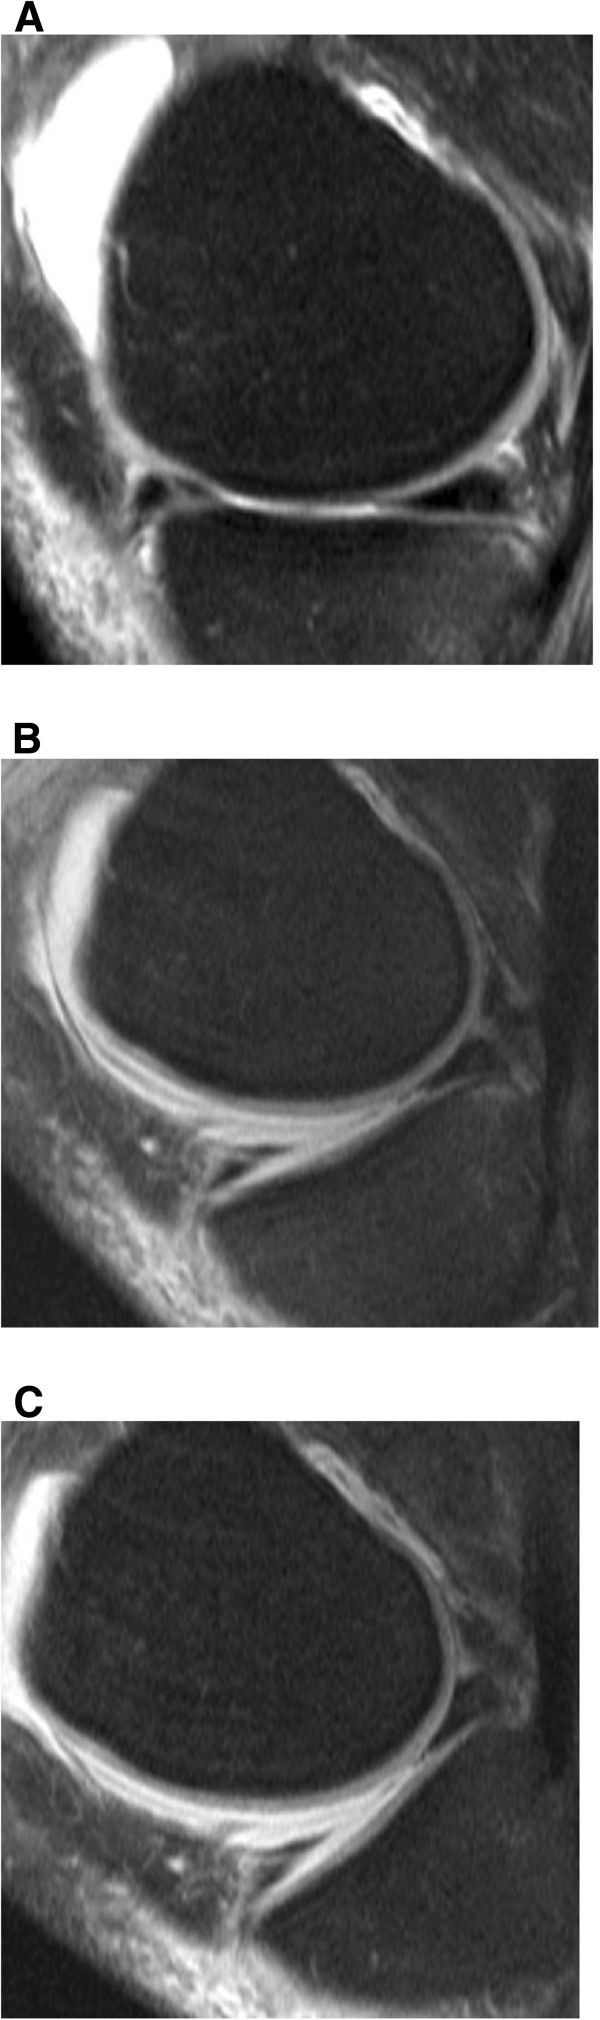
Oblique vertical tear of the anterior horn of the medial meniscus. T2-weighted TSE FS sagittal image (2900/90) obtained at knee extension (A), 30° of knee flexion (B) and 55° of knee flexion (C) demonstrate the oblique vertical tear extending from the surface to the undersurface of the medial menisci. No differences are recognized in the delineation of the tear at all three knee positions.
